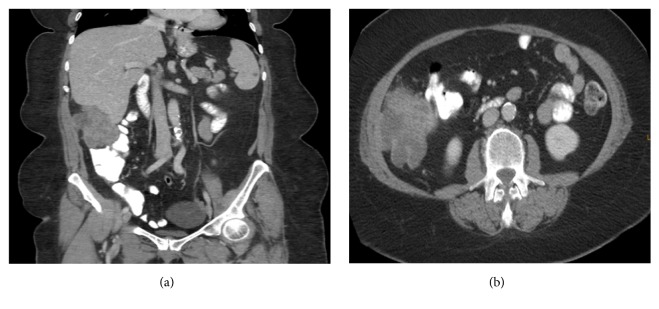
(a, b) CT abdomen/pelvis findings.
